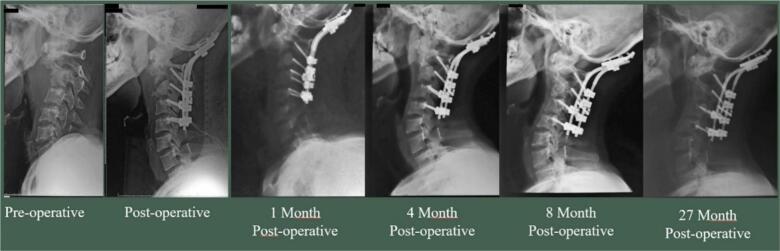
Consecutive X-rays from pre-operative to 27 months post-operative. Shows occipital-cervical fusion with the implant remain intact and was in proper position (red arrow). (For interpretation of the references to color in this figure legend, the reader is referred to the web version of this article.)
